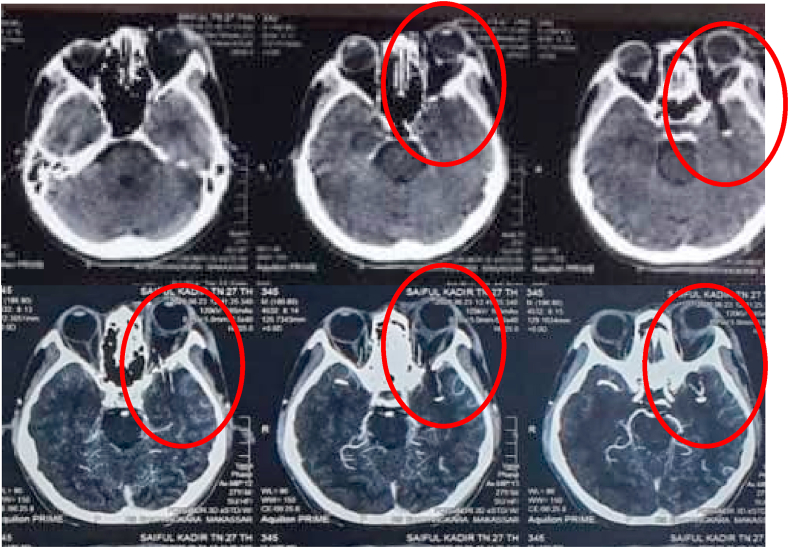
Noncontrast and contrast head CT scan showed a linear-shaped hypodense foreign object in left medial orbit penetrate to the left temporal fossa, no contusion and cerebral edema.
